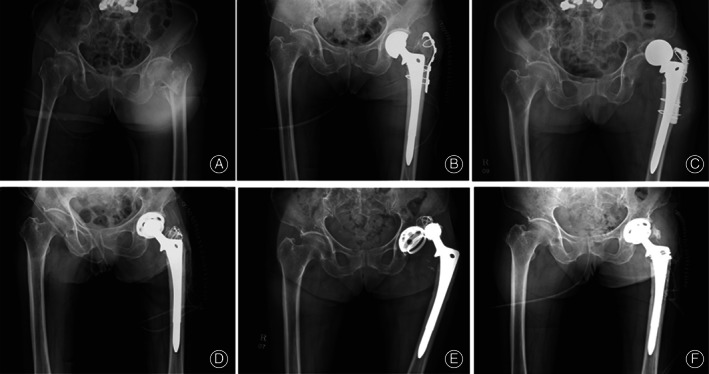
A 71‐year‐old woman who underwent abductor muscle reconstruction using an allo‐Achilles tendon to treat abductor muscle deficiency. (A) Initial preoperative anteroposterior (AP) hip radiograph. (B) Postoperative AP hip radiograph immediately after bipolar hemi arthroplasty (BPHA). (C) AP hip radiographs 3 months after BPHA (dislocation state). (D) Postoperative AP hip radiograph after revision total hip replacement arthroplasty (THRA) using constrained acetabular liners (CAL). (E) AP hip radiographs 2 years after revision THRA (dislocation state). (F) AP hip radiographs after re‐revision THRA with abductor muscle reconstruction using an allo‐Achilles tendon.
